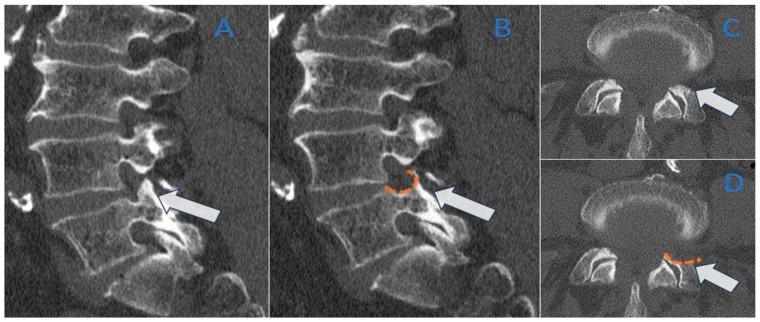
Foraminal stenosis L4–L5; A 57-year-old patient presenting with left lumbar-radicular pain at the L4–L5 level. The preoperative CT images, reconstructed in both sagittal and axial planes with a 1 mm bone window, reveal the presence of a stenotic foramen (arrows), particularly in the postero-inferior aspect, where sclerosis and hypertrophy of the pedicles are evident in images (A,C). Post-treatment (images (B,D), dashed orange lines), there is a clear widening of the foramen in the posterior and inferior sectors, with resolution of the hypertrophic and sclerotic components, and a notable recovery of both the foramen area (B) and transverse diameter (D).
